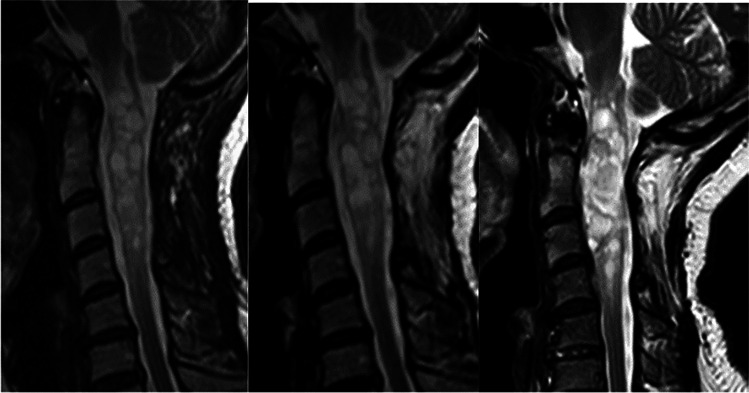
Intramedullary cystic ependymoma. T2 weighted MRI preoperatively, and 3 and 11 years postoperatively
